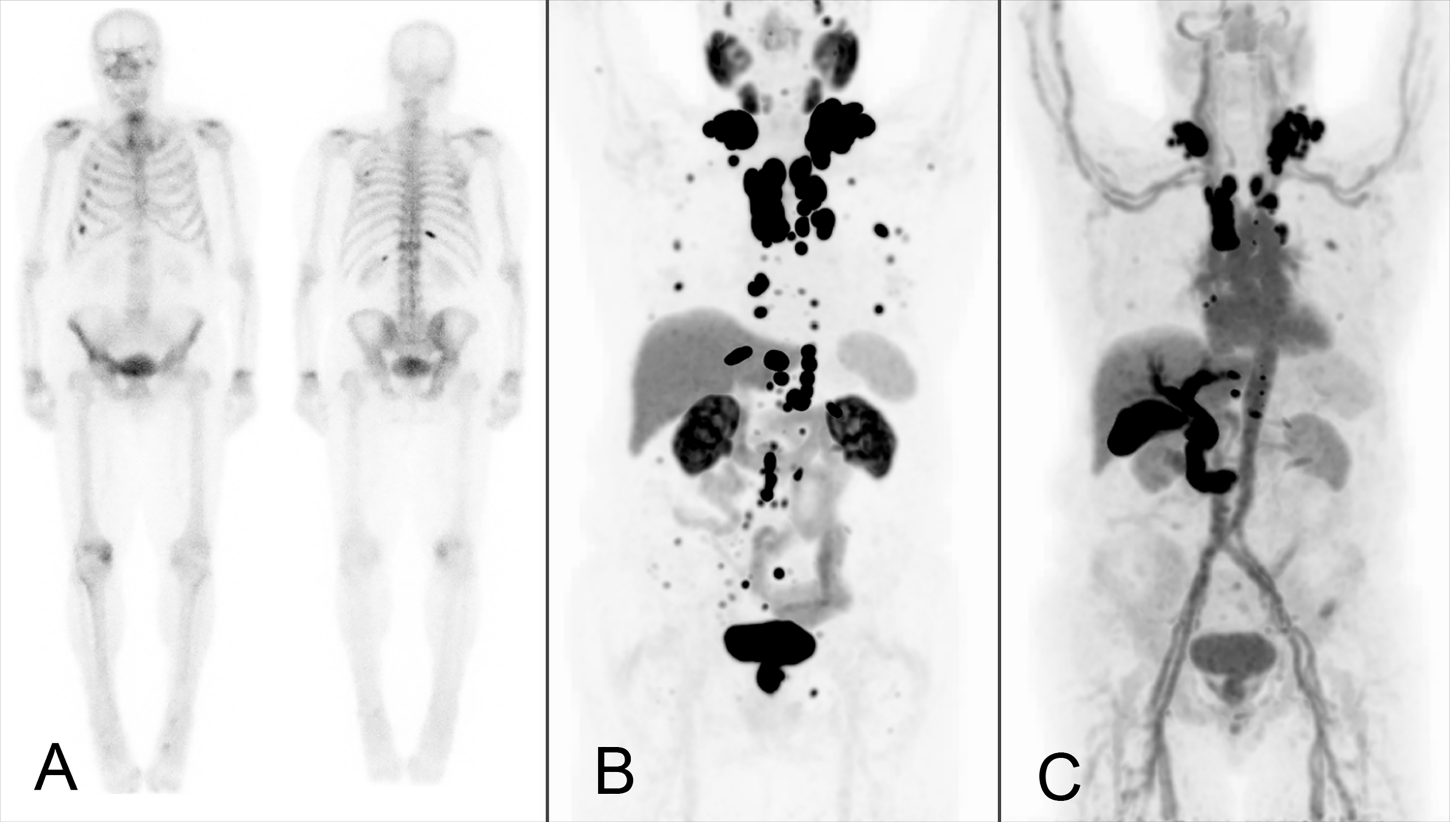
Example of a male patient with bone and lymph node metastases from castration-resistant prostate cancer who underwent bone scintigraphy (A) and ^18^F-DCFPyL (B) and ^18^F-FDHT PET (C) for research purposes. Bone scintigraphy demonstrated several rib metastases. A large number of additional (measurable) bone metastases were observed on ^18^F-DCFPyl PET, with additional lymph node metastases detected on-par. Disconcordant AR-expression visualized on ^18^F-FDHT PET.
